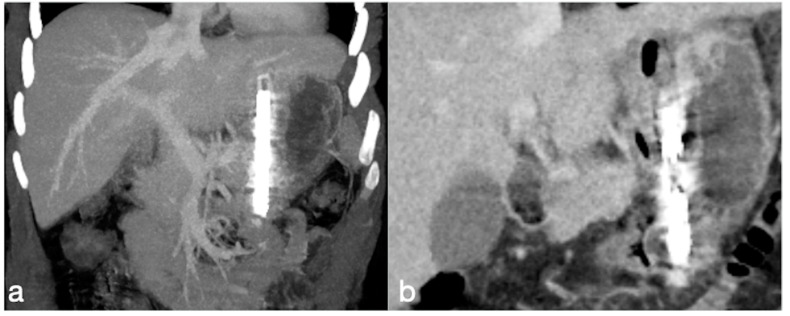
A correct positioning of BariClip^®^ seen at CT coronal MIP (a) and MPR (b) views.
